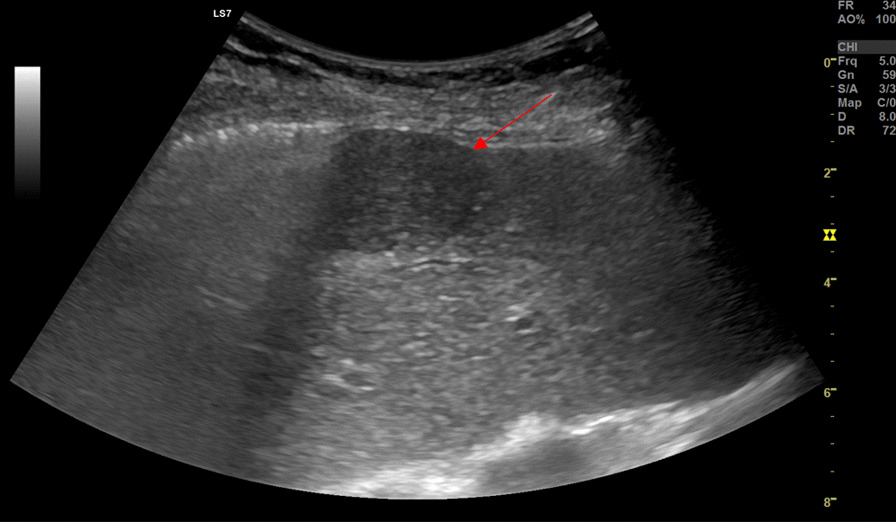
Abdominal B-Mode ultrasound of the hepatic subcapsular hypoechoic nodule. The arrow is pointing to the hepatic subcapsular hypoechoic nodule
